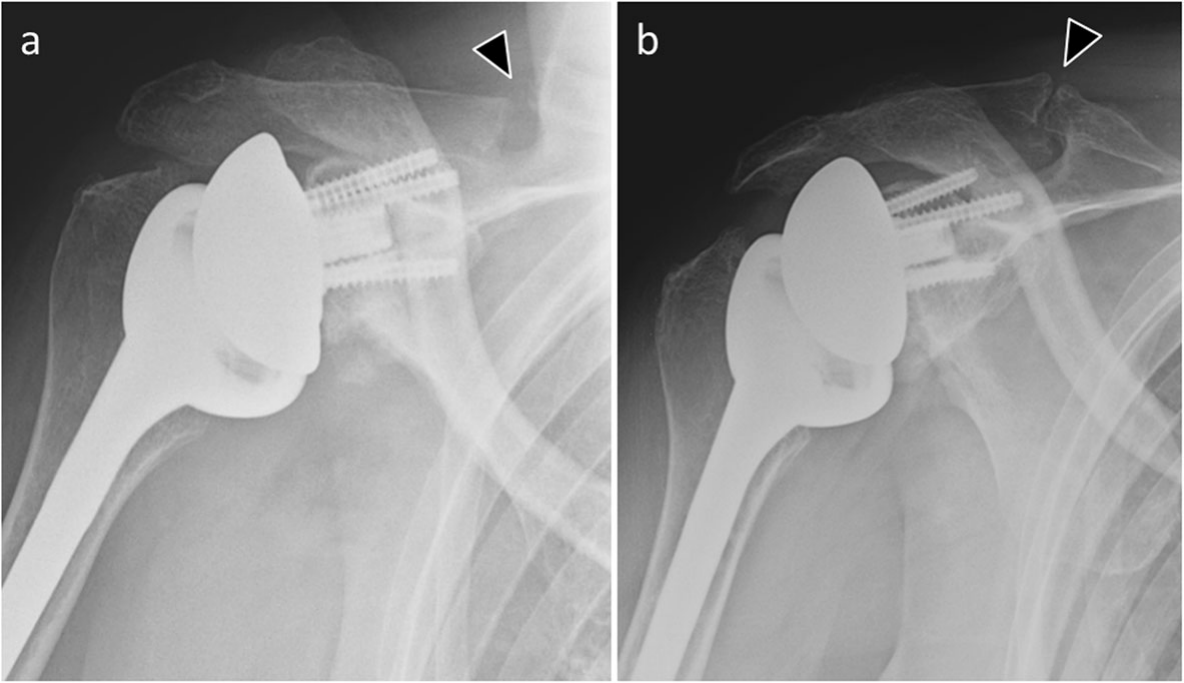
Anteroposterior radiographs of a 67-year-old woman with reverse shoulder arthroplasty. a Radiograph shows a proximal scapular spine fracture (arrowhead). b This fracture is more visible after a few weeks due to pseudoarthrosis (arrowhead)
